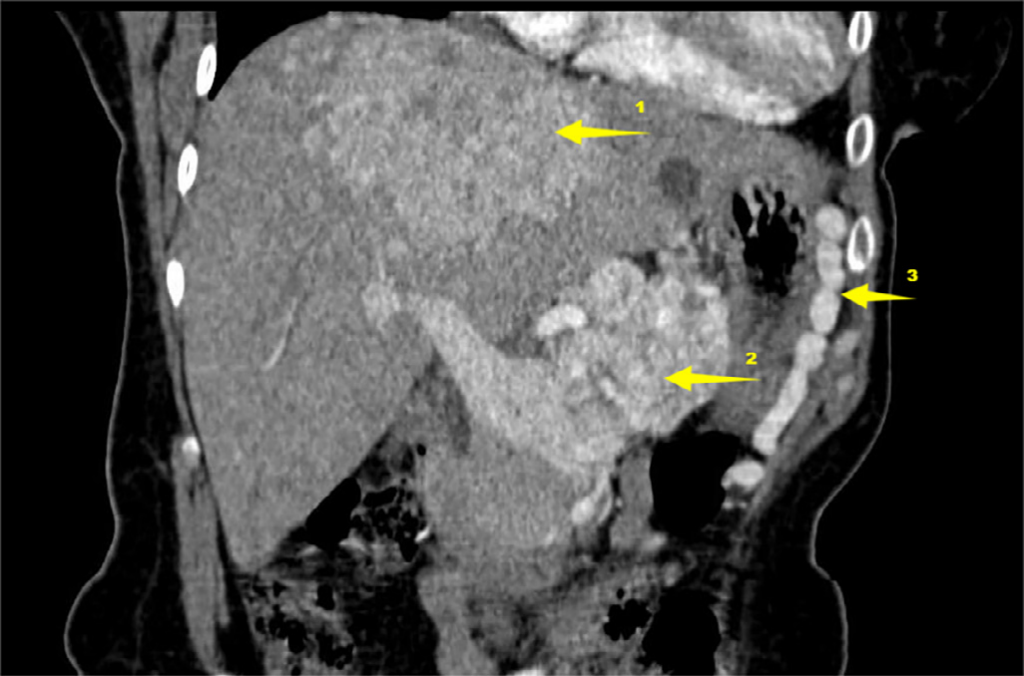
Abdominal injected CT on coronal plane. 1 liver tumor. 2 tumoral thrombosis inside portal vein containing multiple small hyperdense vessel, giving the vessel in vessel appearance. 3 collateral circulation.
